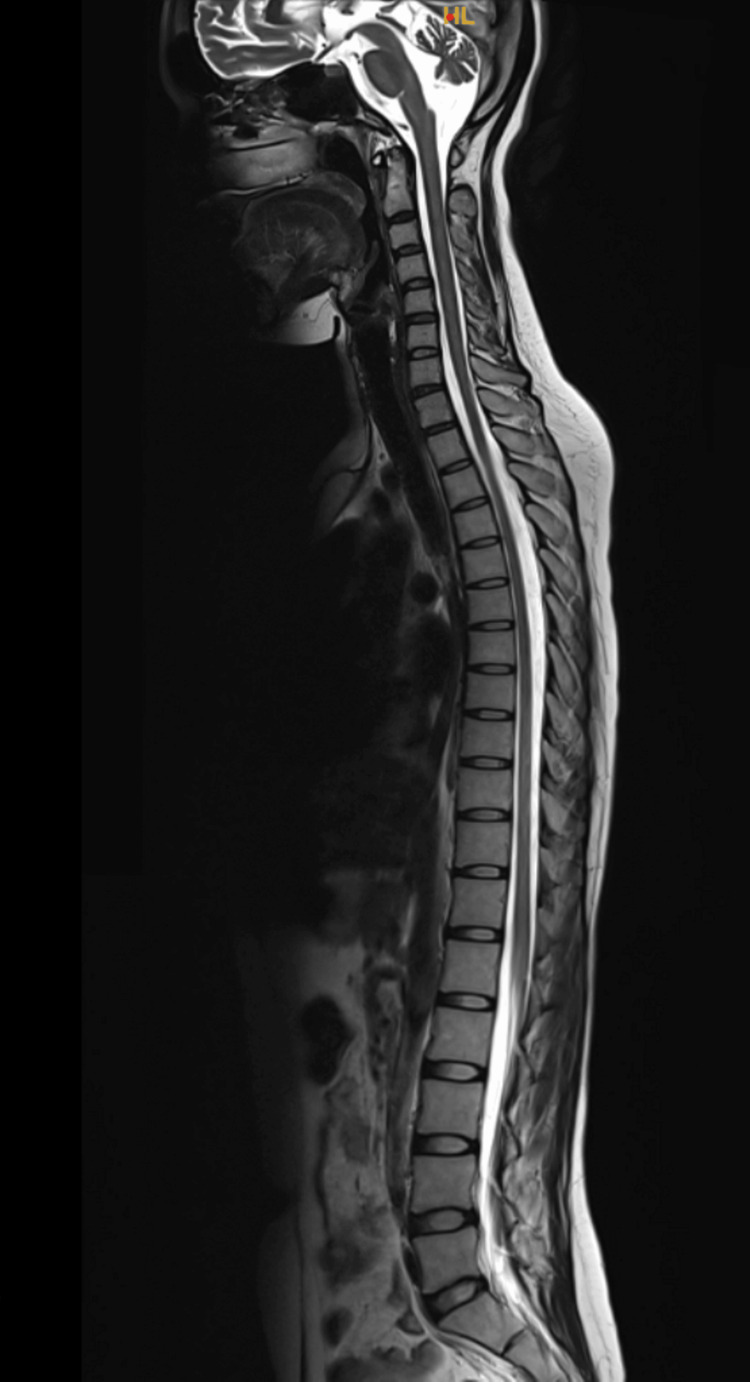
MRI of the spine, which was normal
